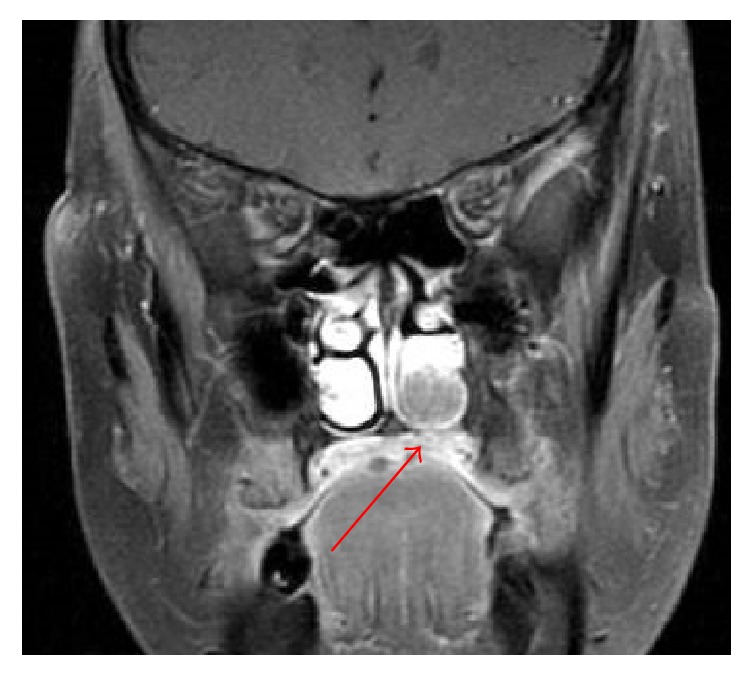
An MRI image shows a tumor (arrow) attached to the left inferior nasal turbinate.
